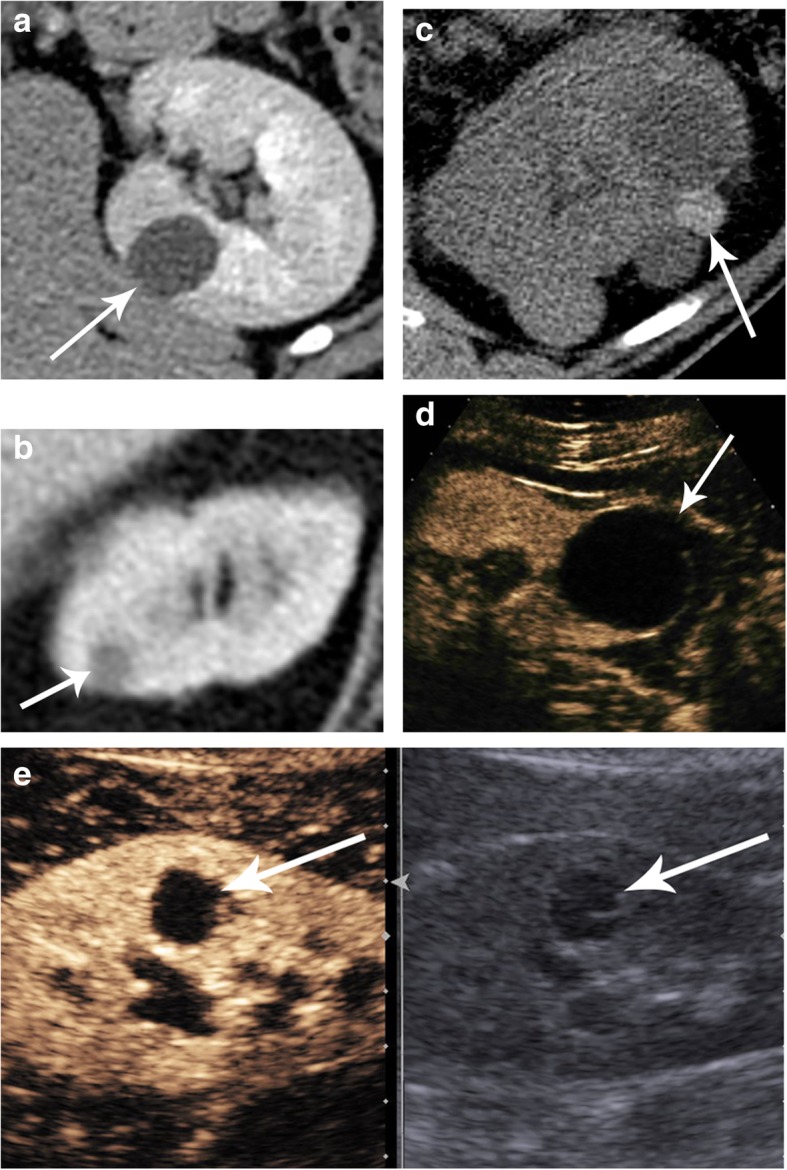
Renal masses that do not need to be followed. a Axial contrast-enhanced CT showing a right renal too small to characterize mass (TSTC) (arrow); the renal mass is 8 mm in size, and the CT slice thickness is 5 mm, making the renal mass size less than twice the reconstructed CT thickness (8 mm/10 mm). b Axial contrast-enhanced CT showing a hypodense left kidney lesion measuring 3 HU (less than 20 HU), which is likely a simple cyst (arrow). c Axial non-enhanced CT showing a hyperdense left kidney cyst with an attenuation value of 85 HU greater than 70 HU, consistent with a hemorrhagic cyst (arrow). d Contrast-enhanced US of a Bosniak I cystic lesion showing no wall thickening or septa (arrow). e Unenhanced (right) and contrast-enhanced (left) US of a renal Bosniak II cystic lesion with thin septa that did not enhance in the post-contrast study (arrows)
